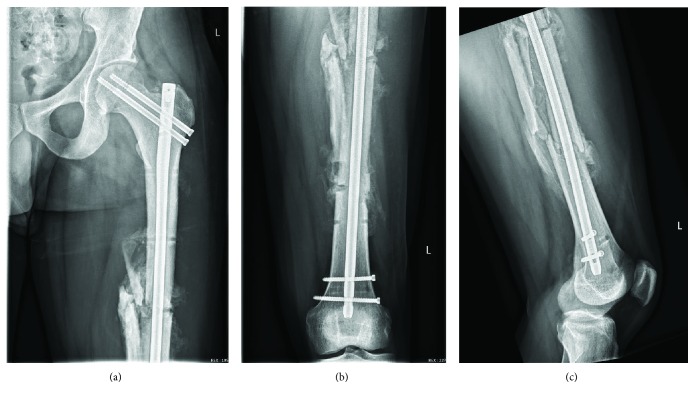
Postoperative anteroposterior radiograph of the left femur (a, b); postoperative lateral radiograph of the left knee (c).
